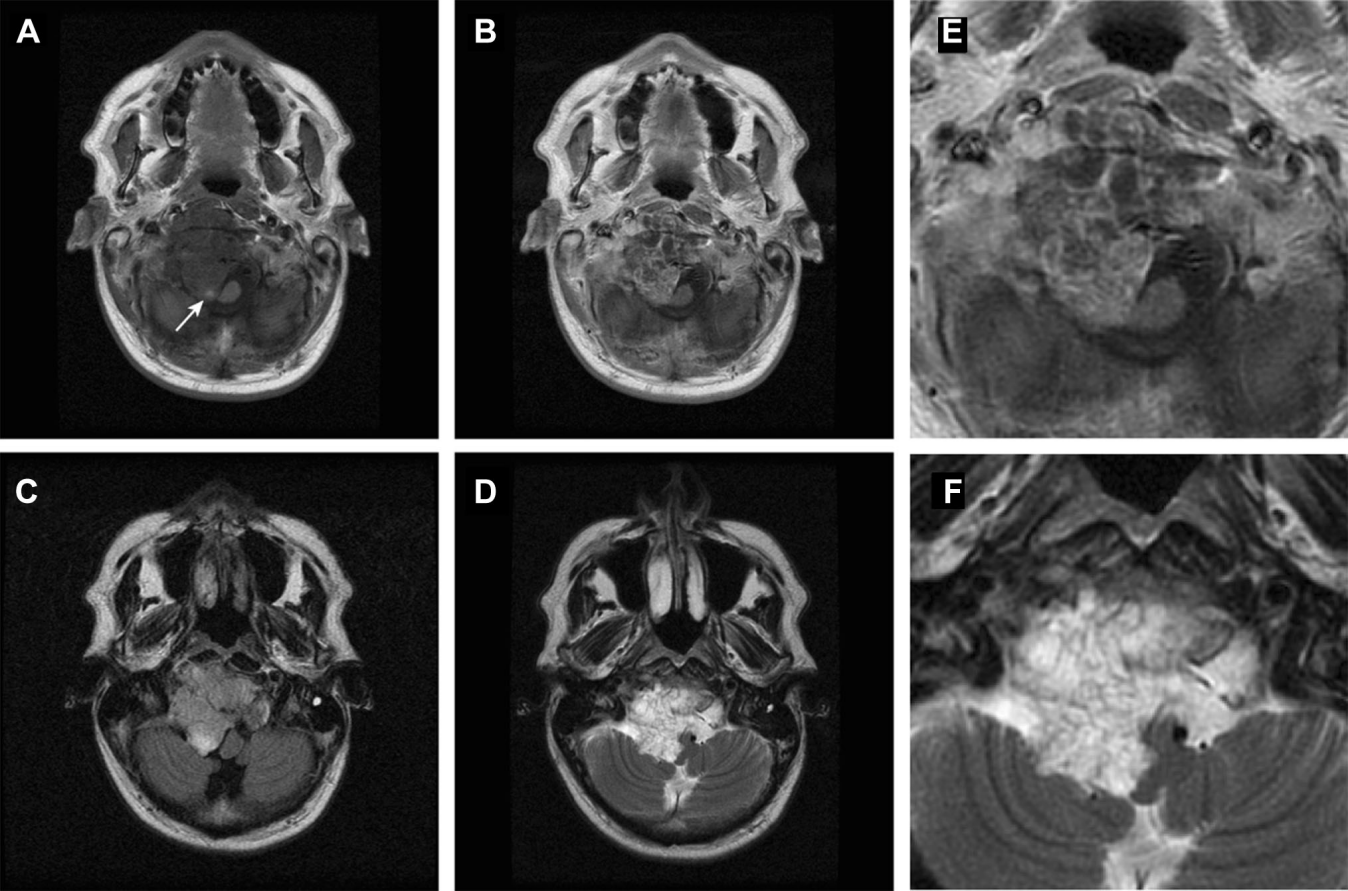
Chordoma. Magnetic resonance imaging of a skull base chordoma arising at the clivus with compression of the brain stem. Axial T1-weighted imaging (a) shows a mass with mostly hypointense signal, a hyperintense focus (arrow) and heterogeneous contrast uptake (b, e). Axial FLAIR (c) shows heterogenous tumour intensity. T2-weighted imaging (c, f) shows mostly hyperintense signal intensity with linear foci of hypointensity. Pathologic examination confirmed the diagnosis of chordoma
